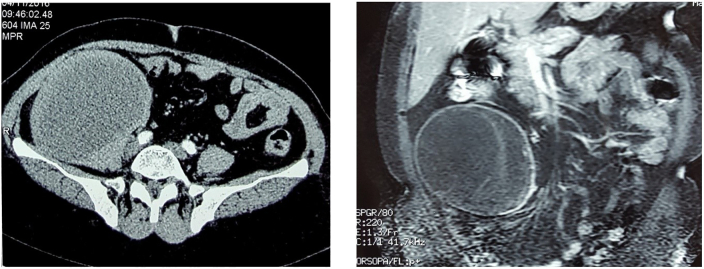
CT imaging showing the right retroperitoneal cystic mass.
